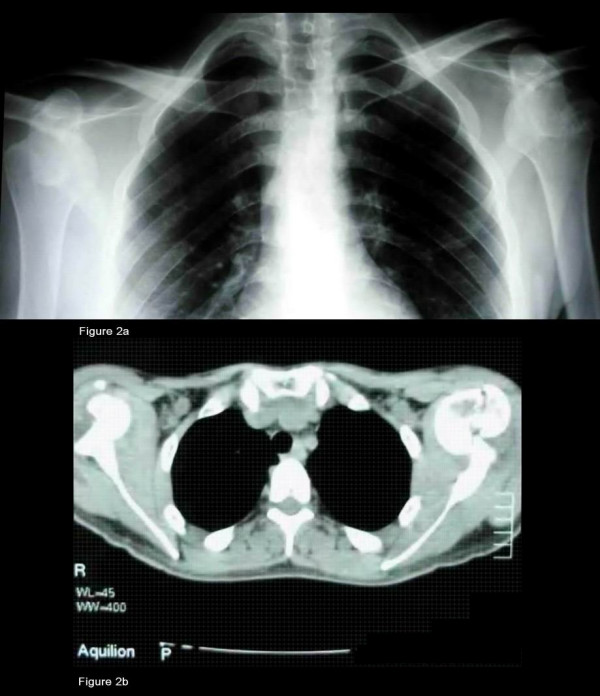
A) Radiographic control before reduction.B) CT scan pre reduction and ORIF (Open Reduction & Internal Fixation) showing a great tuberosity fracture of the left humerus and Hill Sachs lesions at both sites.
